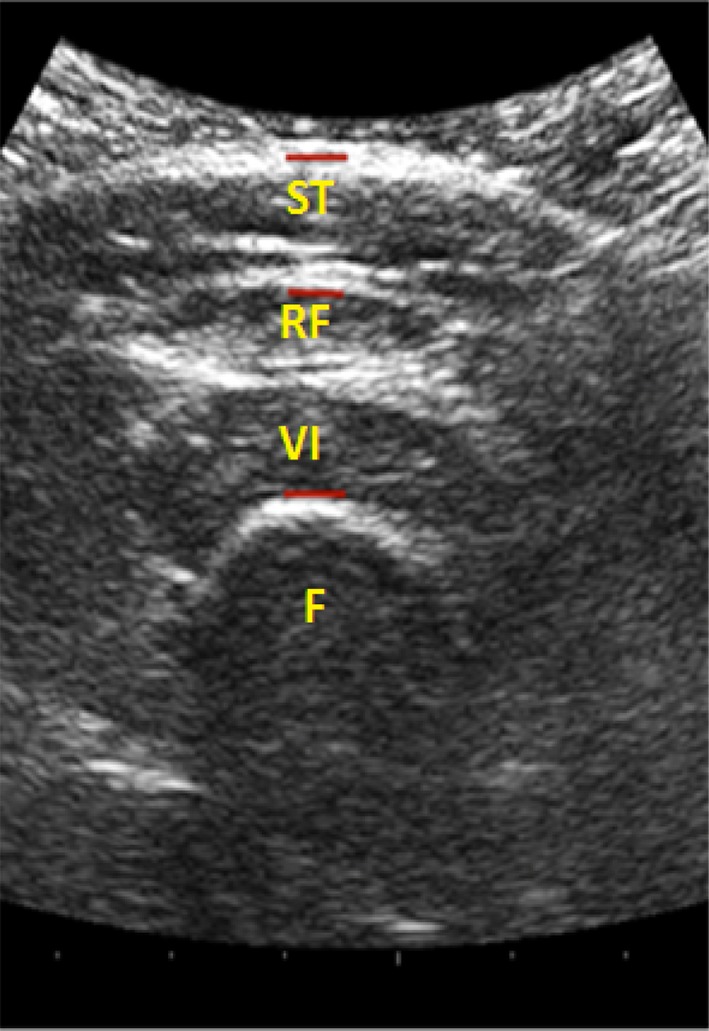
An example of an ultrasound image. Subcutaneous tissue (ST) comprising superficial layers (fat and fascia), and rectus femoris (RF) and vastus intermedialis (VI) also known as deep layers (quadriceps muscle), and femur (F)
